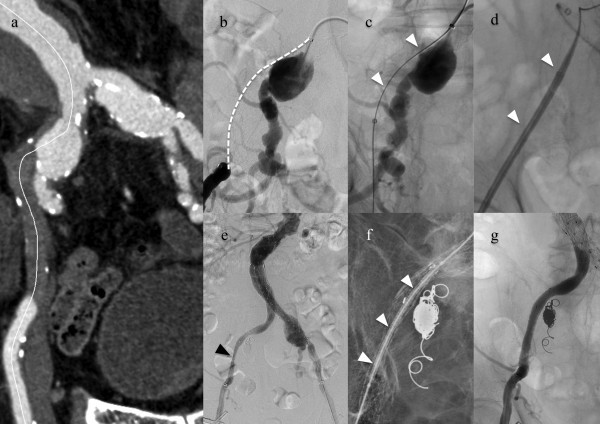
An example of the procedural steps. (Case 1), a. Curved multiplanar reconstruction of the CTA showed AAA, bilateral CIAA and right EIA occlusion. b. Angiogram showed CTO of the right EIA (dashed line) and right CIAA. c. Lesion was successfully crossed using bidirectional wiring (white arrow head). d. After predilation, a 14-Fr sheath was advanced along the wire (white arrow head). e. Completion angiogram showed residual stenosis at the right EIA despite placement of a self-expanding stent (black arrow head). f. Express LD stent was deployed to support the self-expanding stent (white arrow head). g. Successful dilation of the EIA was achieved. AAA: abdominal aortic aneurysm, CIAA: common iliac artery aneurysm, CTO: chronic total occlusion, EIA: external iliac artery.
